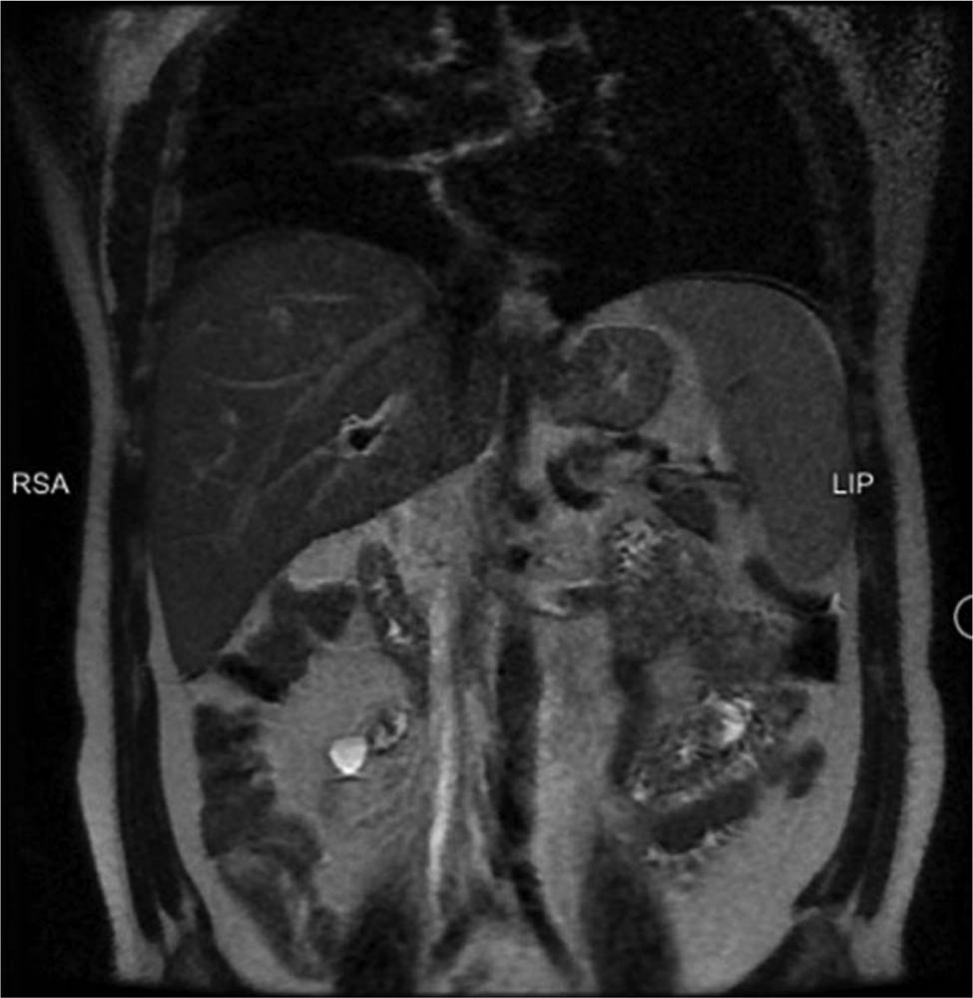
Coronal MRI showing irregularity of the liver border indicating early stage of cirrhosis (black arrowhead).
